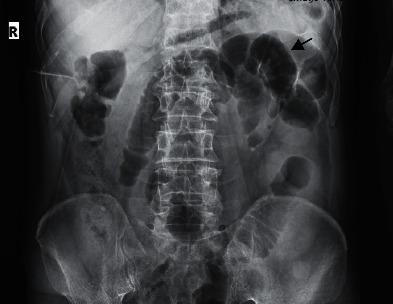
Male patient's supine abdominal X-ray.
